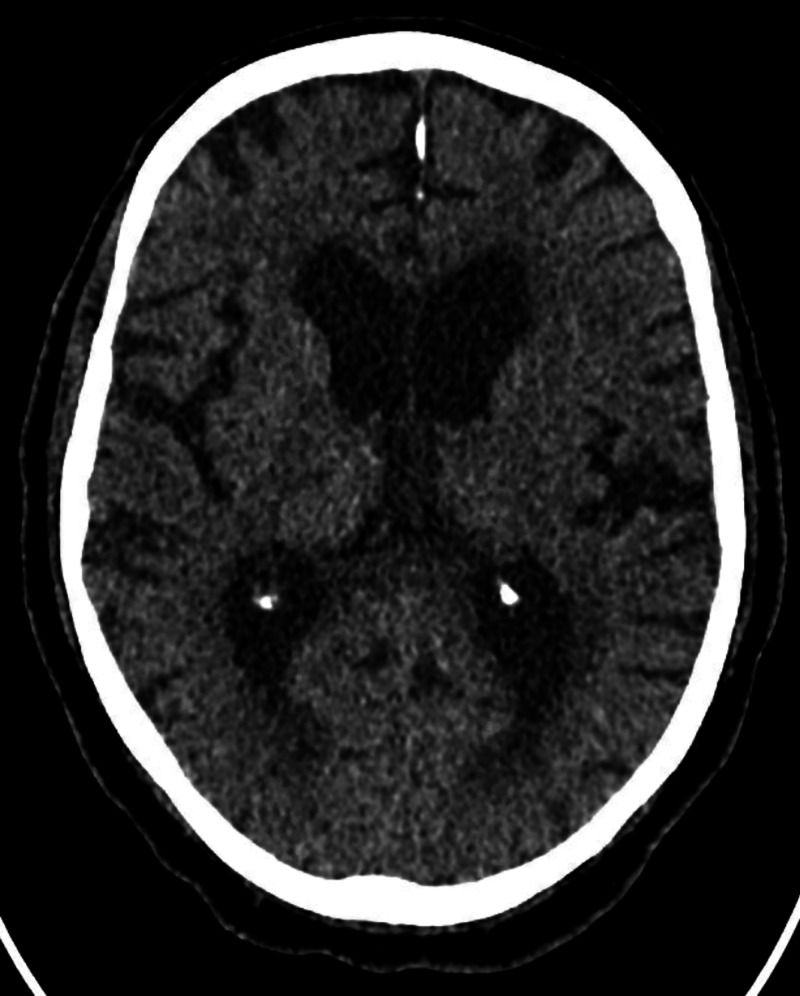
CT head without contrast showing no acute intracranial bleed or ischemia
